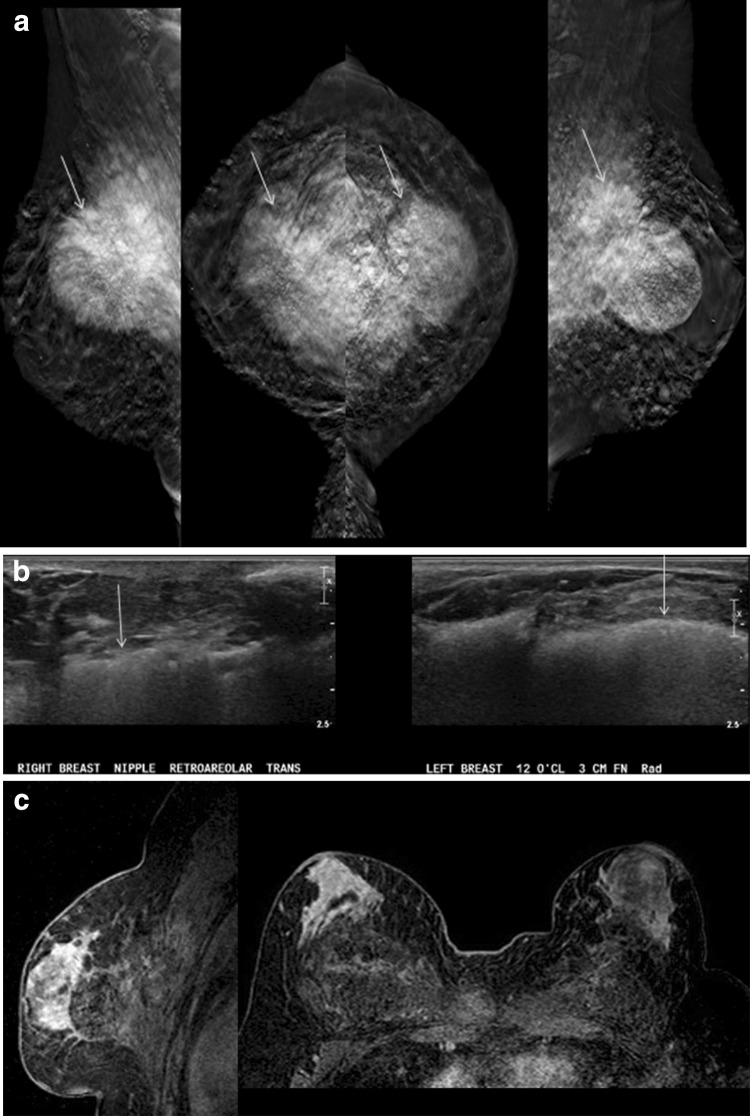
A 54-year-old transgender woman is referred for a screening mammography prior to nipple sparing mastectomy to remove painful masses caused by free silicone. a Tomosynthesis shows bilateral large dense masses which are inseparable from the pectoralis muscle (arrows). There are also innumerable small silicone granulomas. b Ultrasound shows an example of the indistinct hyperechoic “snow storm” appearance of free silicone at posterior depth in both breasts (arrows). c Post-contrast subtracted MRI image shows no suspicious enhancing mass
